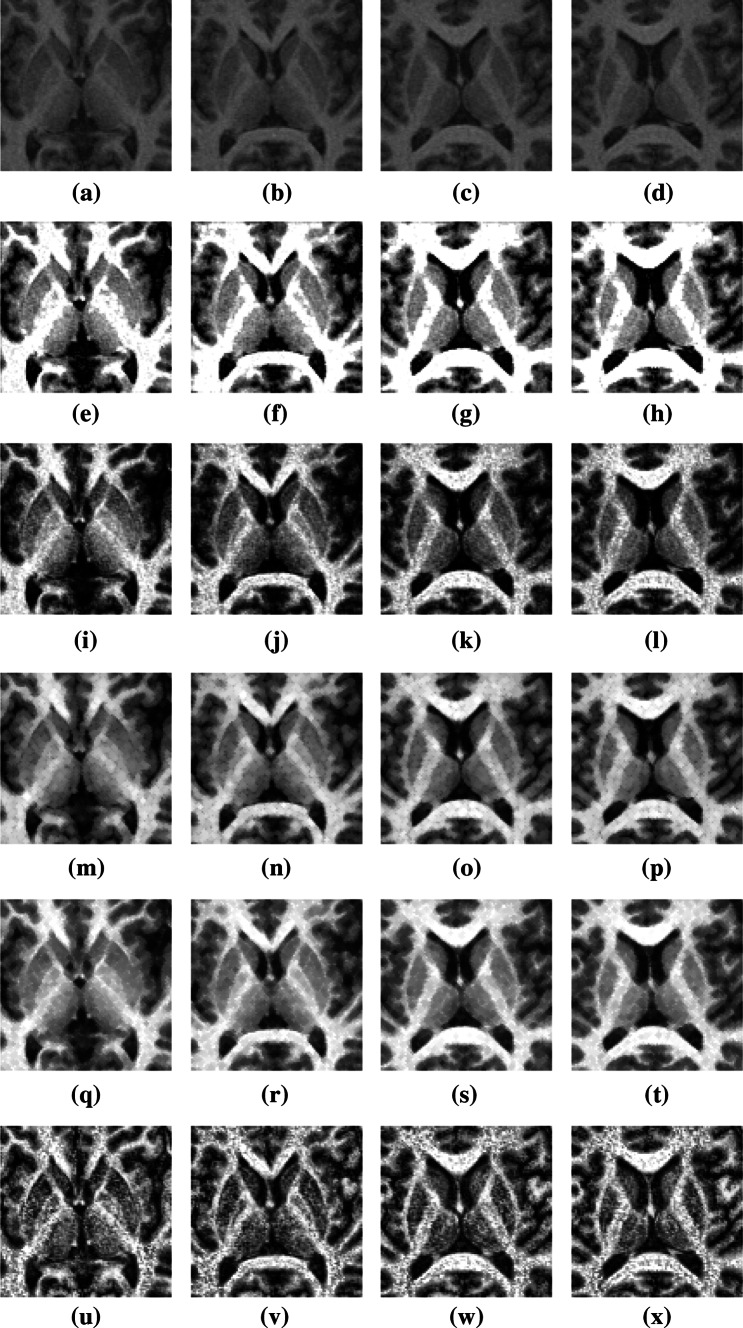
a–d MRI images of patient 1, e–h results obtained using proposed method, i–l HE images, m–p opening images, q–t closing images, and u–x CLAHE images
